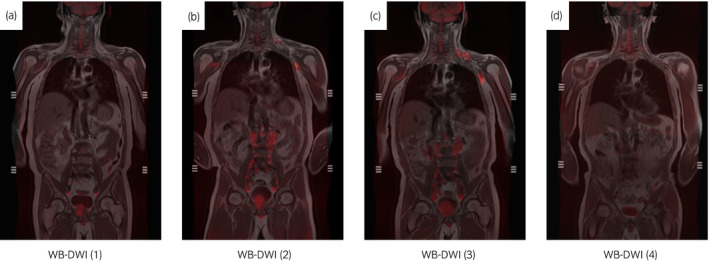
WB‐DWI findings before and after EP chemotherapy. Images are fusion images of DWI signals (shown in red) and morphological images (T1‐weighted images).
